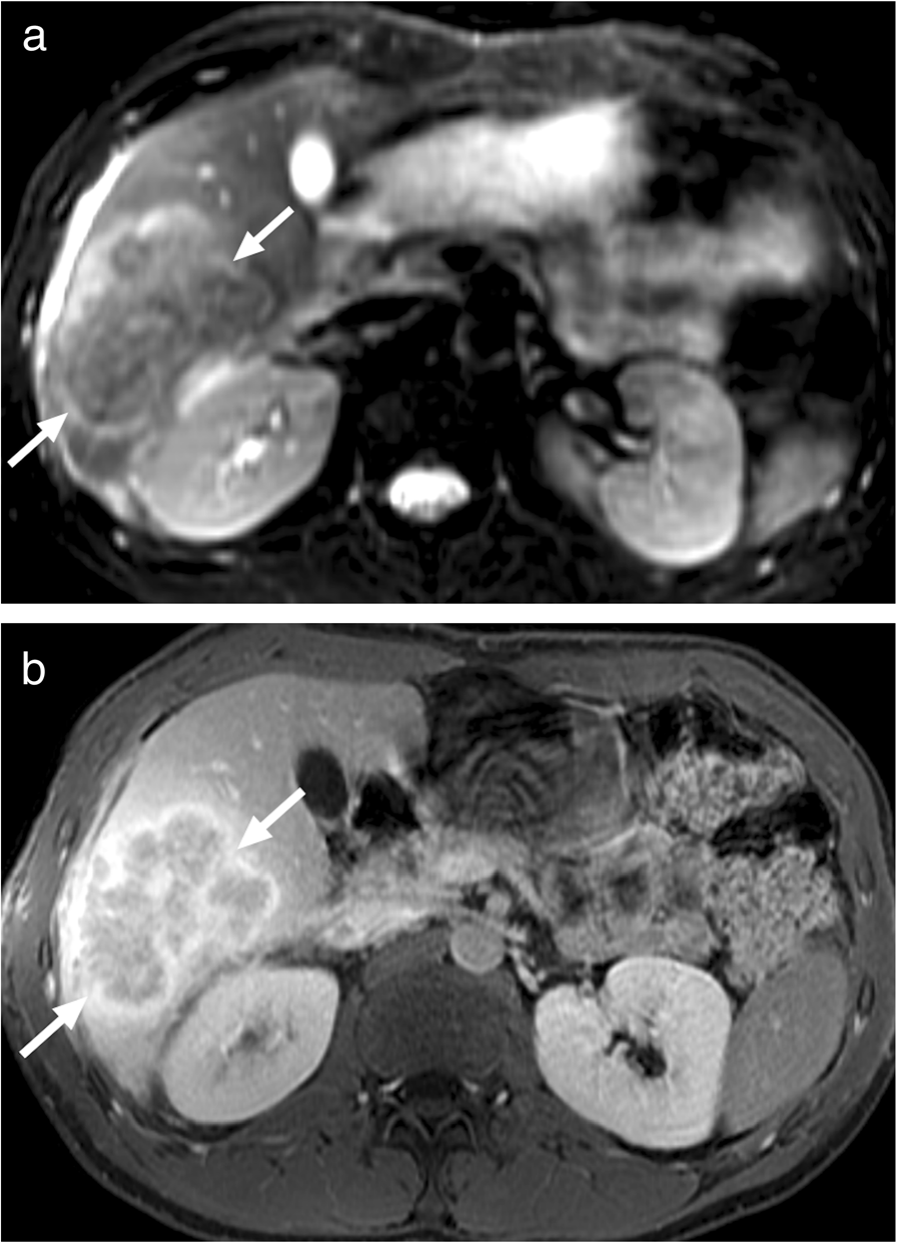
A 28-year-old male with no significant medical history presented with right upper quadrant pain and weight loss. a T2W axial plane MR image demonstrates a solid mass (arrows) within the right liver lobe. b Postcontrast axial plane T1W image in the portal venous phase demonstrates heterogenous enhancement of the lesion (arrows). Surgical resection, with a putative diagnosis of cholangiocellular carcinoma, of this mass revealed inflammatory pseudotumor with features suggestive of IgG4-related sclerosing cholangitis. Post-surgical evaluation revealed elevated serum IgG4 levels
